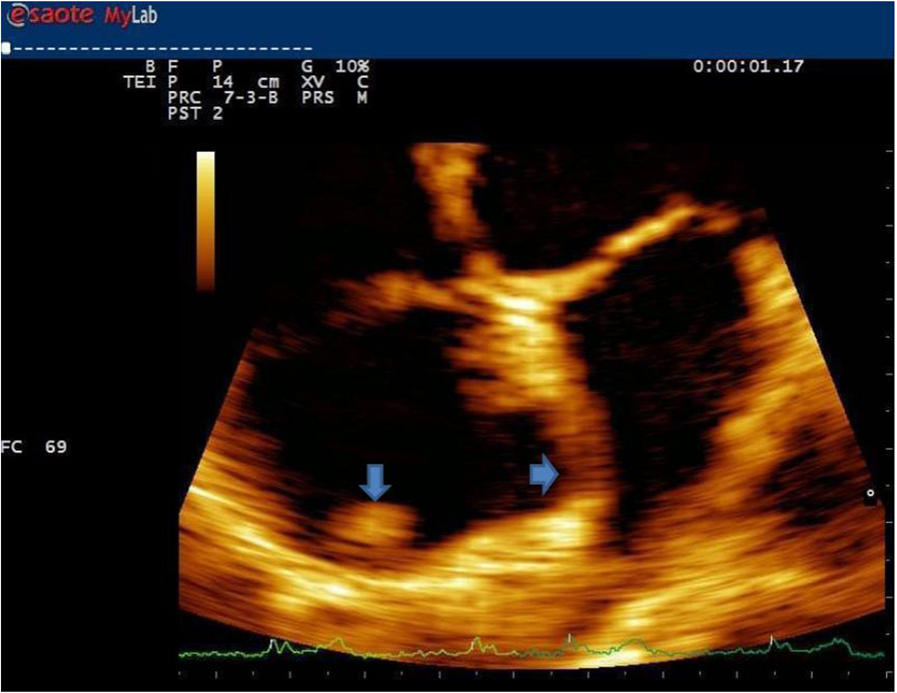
Zoom transthoracic echocardiogram in four-chamber view focusing on crista terminalis and atrial septal aneurysm (arrowheads).
